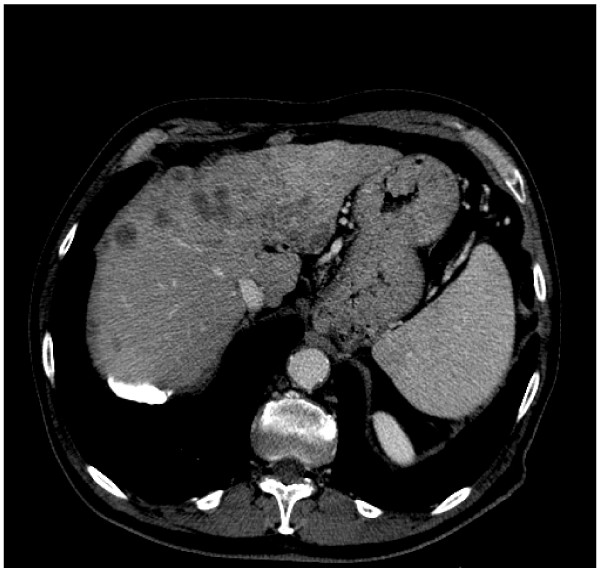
CT-scan of upper abdomen showing multiple hepatic lesions. Pre-treatment hepatic evaluation performed at the time of initial diagnosis by CT-scan showed multiple hepatic lesion ranging from 0.3 to 2.0 cm in both liver lobes
